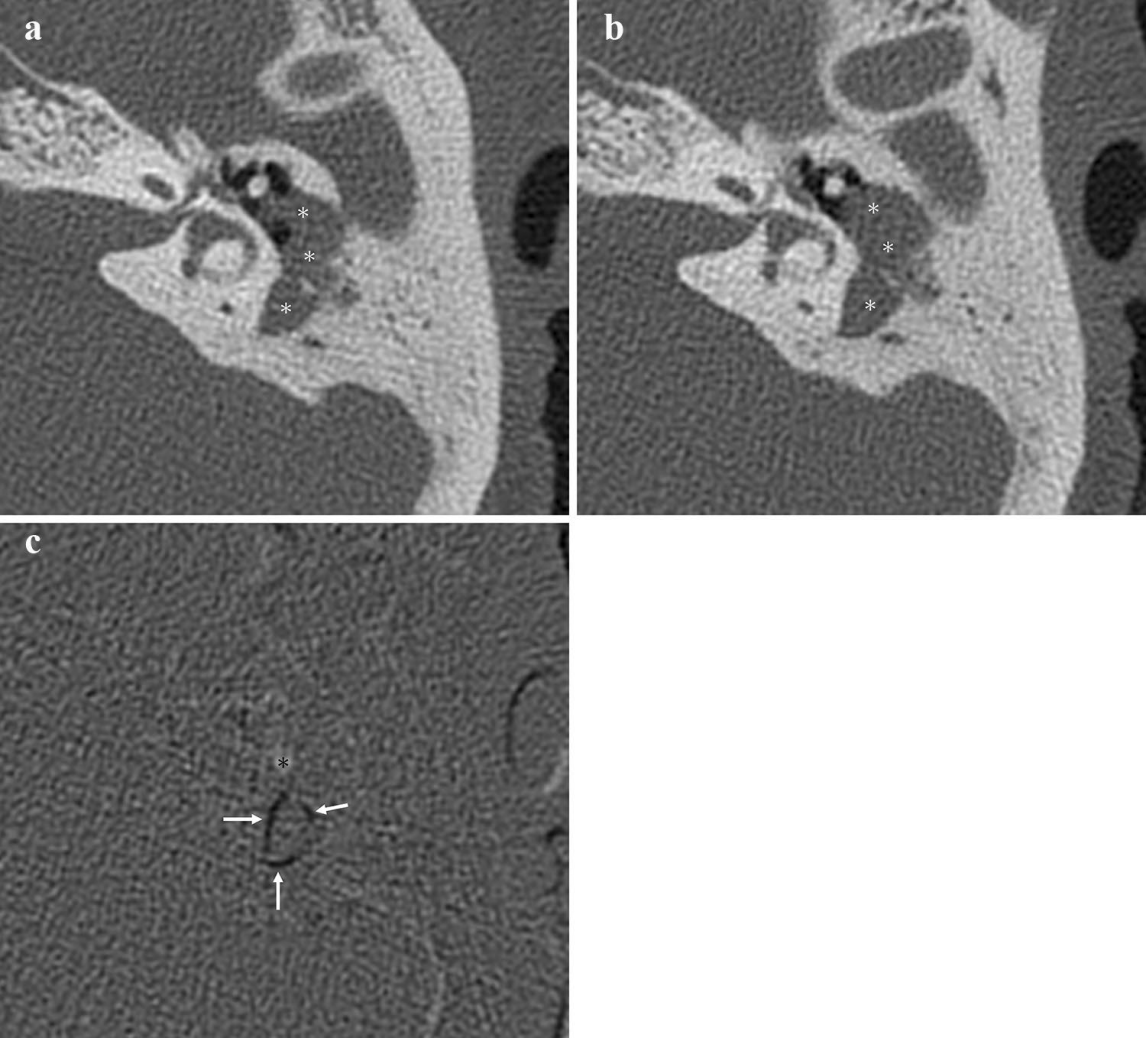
Postoperative residual lesion of left pars flaccida cholesteatoma with relatively slight bone erosive changes. Postoperative temporal bone CT (a) about 1 years ago shows a soft tissue density (*) in the left tympanic and mastoid cavity. Temporal bone CT (b) before surgery for recurrence/residual shows increased soft tissue density (*) and adjacent progressive bony erosive changes (black arrows). In TSCT (c), progressive bone erosive changes are visualized as distinctive black areas (white arrows), and areas of increasing soft tissue density are visualized as white areas (*). TSCT, temporal subtraction CT
